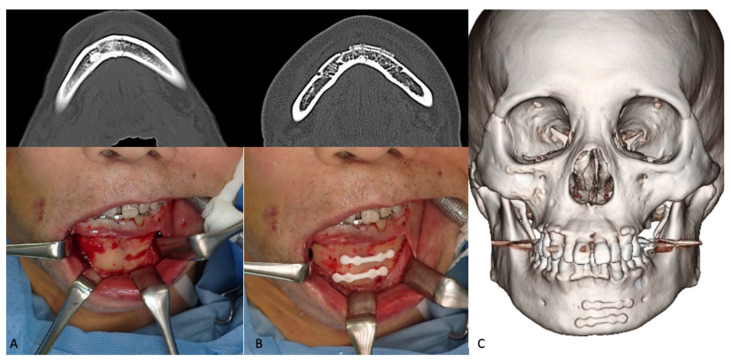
Representative photographs of the two-miniplate fixation technique for mandibular symphysis fractures using the u-HA/PLLA bone-fixation-device system. An 83-year-old man was diagnosed with right orbital floor, right zygomaticomaxillary, and mandibular symphysis fractures. The mandibular fracture was treated using two straight u-HA/PLLA plates. (A), Upper, Preoperative CT scan image; Lower, Exposed mandibular fracture. (B), Upper, Postoperative CT scan image; Lower, Mandibular fracture fixation using two u-HA/PLLA miniplates. (C), Six-month postoperative 3D CT scan.
